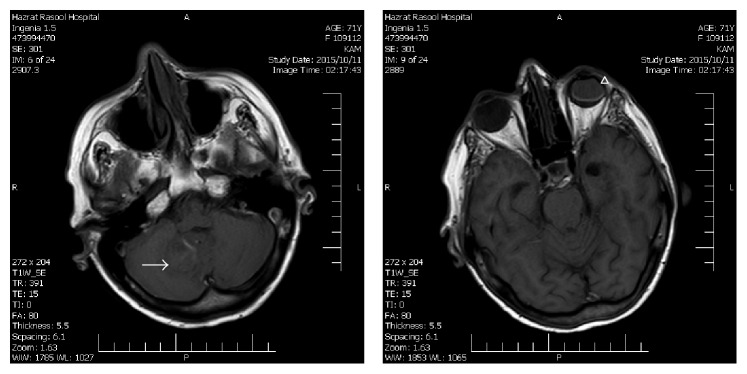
T1-weighted MR images. The intraocular silicone oil (arrowhead) is hyperintense relative to the vitreous of the fellow eye and isointense relative to the cerebellar hemorrhage (arrow).
